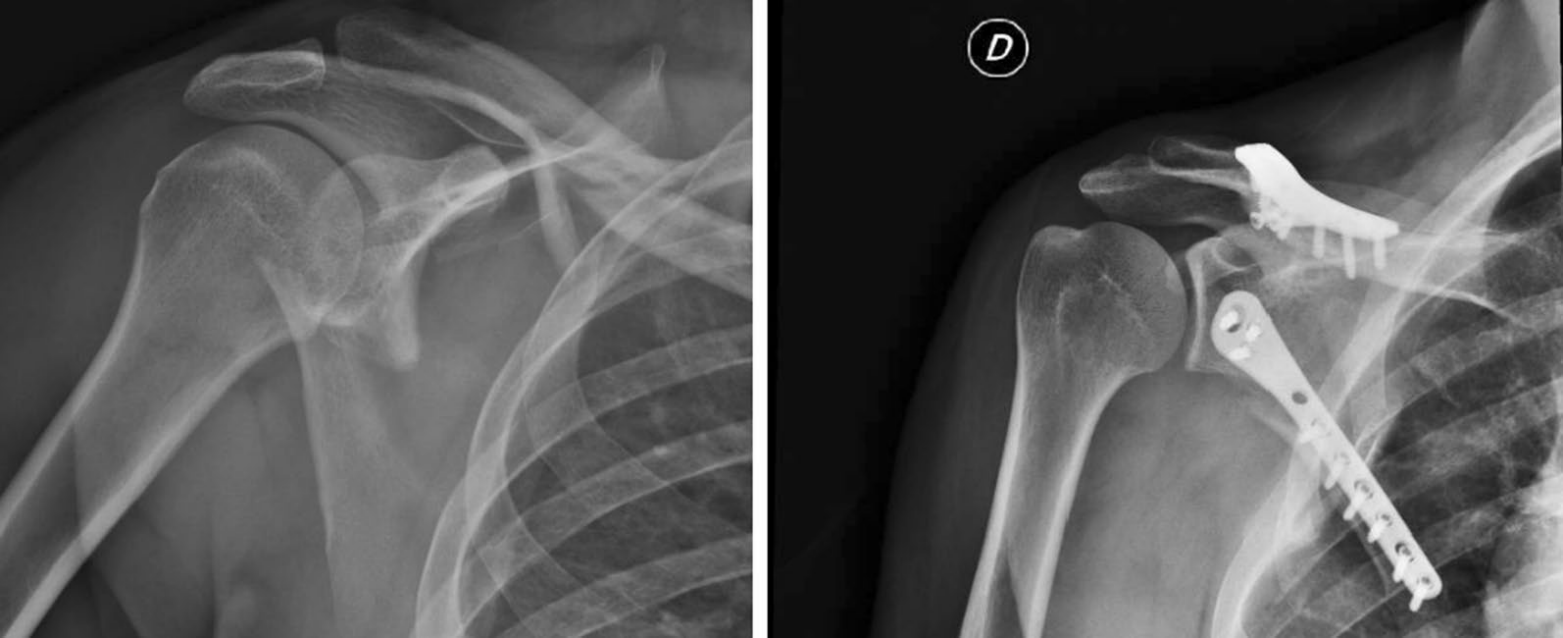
Scapular neck and clavicle fracture (pre- and postoperative X-ray)
